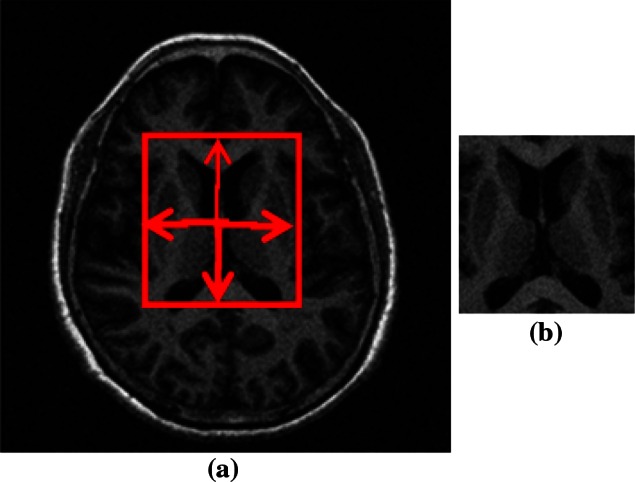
a Original MRI image and b segmented ROI
